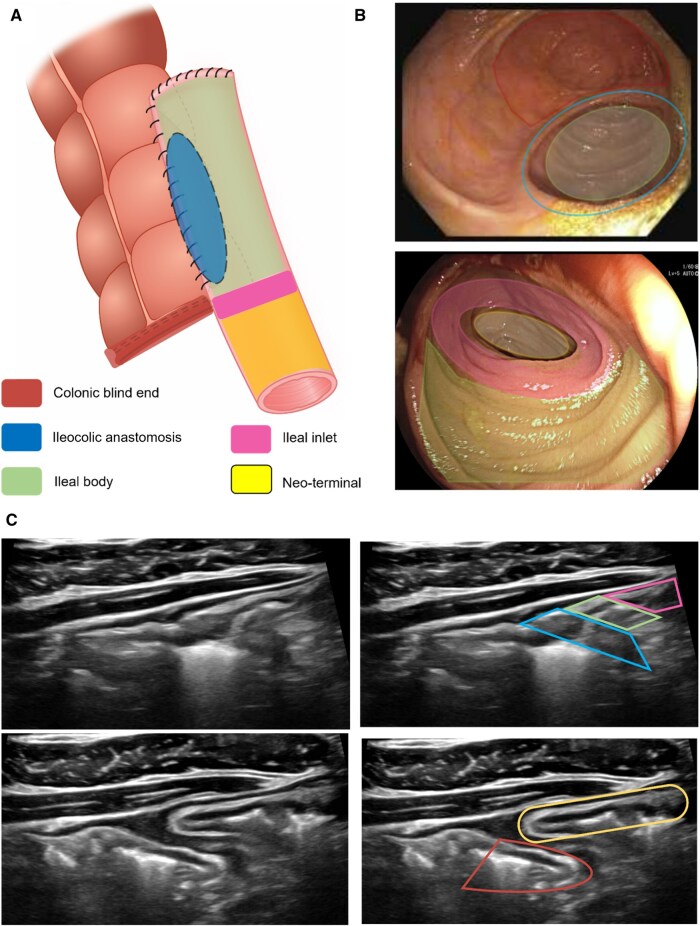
Ileocolic anastomosis landmarks. (A) The anatomic landmarks are highlighted with their corresponding colors on (B) ileocolonoscopy and (C) intestinal ultrasound: colonic blind end (red), ileocolic anastomosis (blue), ileal body (green) ileal inlet (pink), and neoterminal ileum (yellow).
